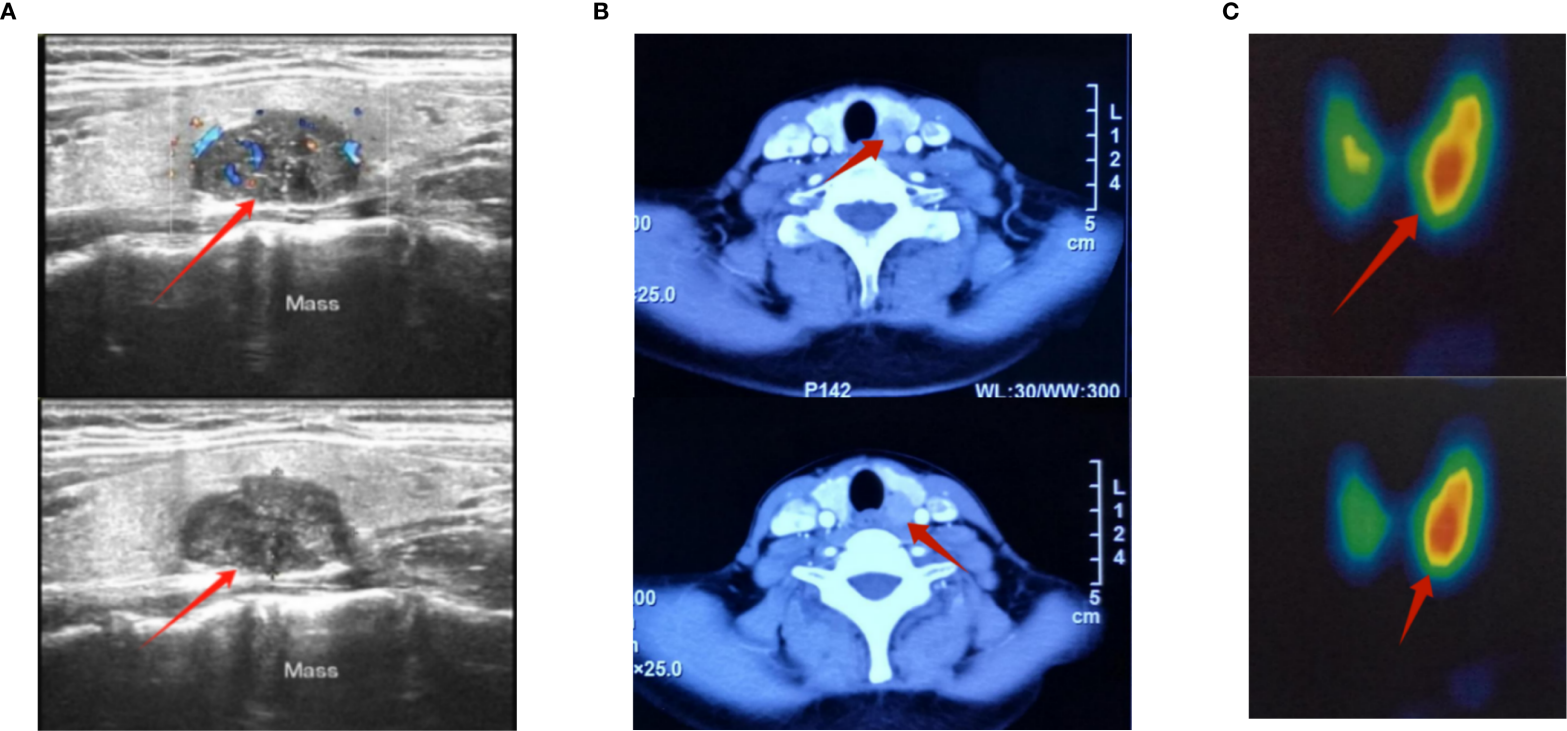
Preoperative color Doppler ultrasound, CT and 2.99mTc-MIBI images of the neck for parathyroid tumor. (A) Preoperative color Doppler ultrasound image of the parathyroid gland; the red arrow marks the location of the tumor. The diagnosis showed a solid mass in the left lobe of the thyroid gland, measuring 27×21×17 mm, of unknown nature. (B) Preoperative CT image of parathyroid gland, the red arrow marks the location of the tumor. The result showed a nodule in the inner and posterior part of the left thyroid lobe, measuring 14×13×17mm, which was considered to be an adenoma. (C) Conventional thyroid imaging before parathyroid surgery 2.99mTc-MIBI thyroid-parathyroid imaging showed: Parathyroid imaging (digital subtraction imaging) showed a radioactive distribution concentration focus in the area equivalent to the left lower parathyroid gland, with a size of about 1.5×2.0cm. There was no obvious abnormality in the position of the thyroid gland, which still maintained a “butterfly” shape. The bilateral lobes were not large in shape, and the radioactive distribution was uneven. The asymmetric uptake rate (%): right lobe = 0.4, left lobe = 0.6, total: 1.0. Area (Cm²): right lobe = 7.9, left lobe = 9.4, total: 17.3. Digital subtraction imaging showed the imaging findings of left lower parathyroid adenoma and hyperfunction; the red arrow marks the location of the tumor.
